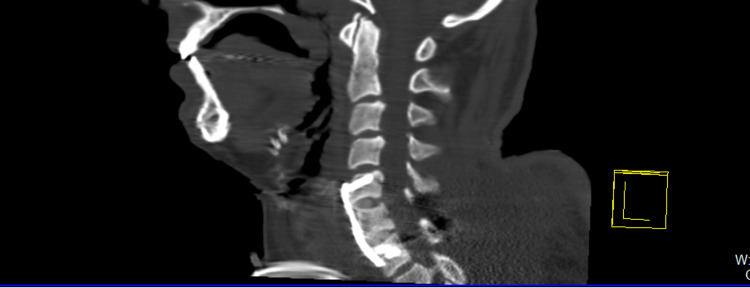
Case 2 CT scan post-surgical sagittal views of the anterior and posterior fixation from C5 to C7, showing the anterior cervical plate, intersomatic tricortical graft, and transfacetary fixation.
